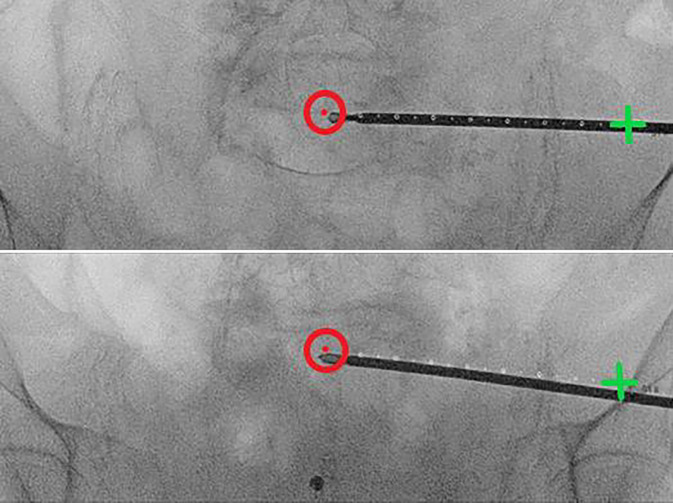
The guidewire position is verified using the predefined personalized inlet and outlet angles (see *appendix* *2*). While alternating between these personalized angles, the guidewire can be repositioned and driven into its final position with the drill. Key to this process is keeping in mind the vital landmark: the slightly S‑shaped anterior cortex of the S1 vertebral body. In uncertain scenarios, a higher dosage x‑ray may be acquired, or the contralateral side extrapolated for reference. Possible mismatch between diagnostic and intraoperative CT may result in an inaccurate planned screw pathway overlay; in such cases, the overlay can still provide an estimated guidewire pathway and end position. After final placement of the guidewire, its length is measured
